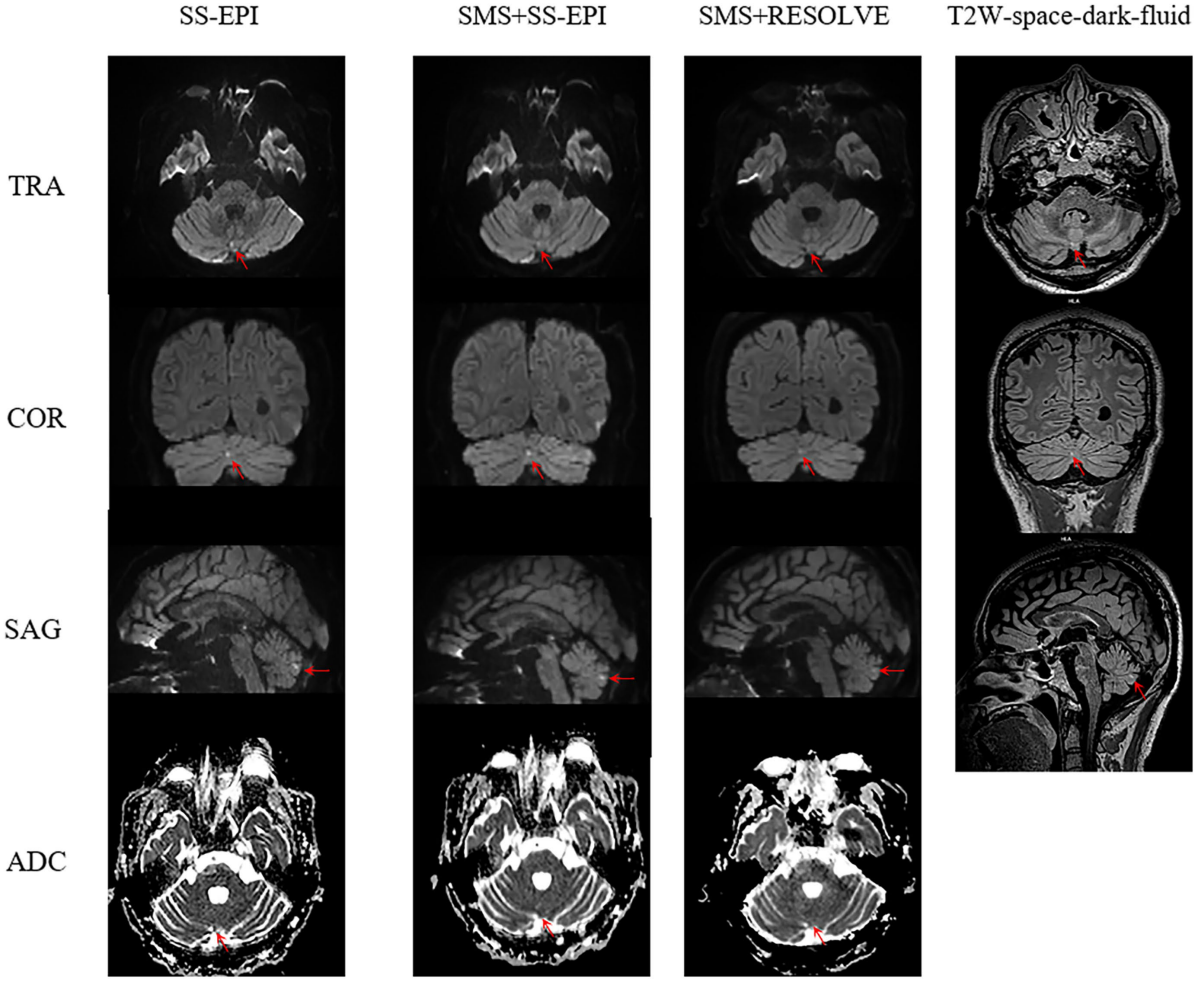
Male, 47 years old. SS-EPI-ISO DWI sequence (red arrow) reveals punctate high signal intensity in the vermis of the cerebellum, with low signal intensity on the apparent diffusion coefficient (ADC), scoring 4 in visibility. SMS + SS-EPI-ISO DWI sequence faintly shows blurry punctate high signal intensity, with low signal intensity on ADC, scoring 3 in visibility. The initial evaluation of the SMS + RESOLVE-ISO DWI sequence did not reveal any abnormal high signal, and retrospective comparison revealed a faint high signal in the vermis of the cerebellum with a visibility score of 1. T2W-space-dark-fluid sequence demonstrates corresponding punctate high signal intensity in the vermis of the cerebellum.
